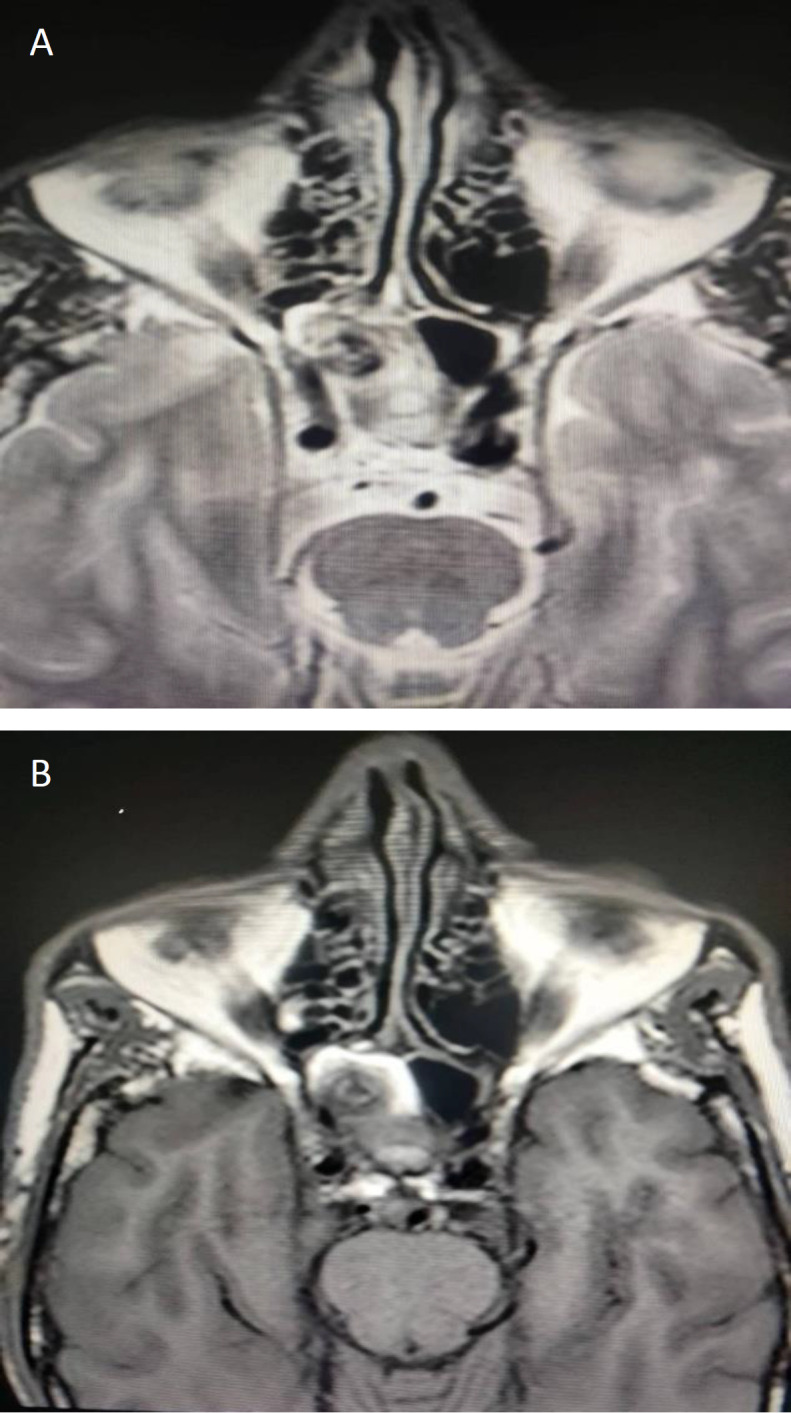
T2W (3A) and T1W (3B) axial MRI scans show a rounded T2 signal void within the sphenoid sinus in continuity with the cavernous segment of right ICA, suggestive of an aneurysm, surrounded by T1 hyperintense and T2 heterogeneously hypointense content, suggesting surrounding hematoma.
